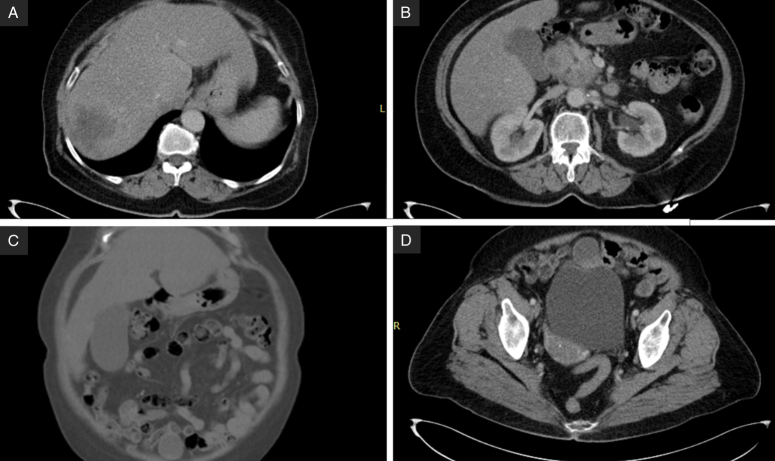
Computed Tomography scan, (A) Axial section: The seventh hepatic segment mass appearing hypodense, (B) Axial section: 53×77 mm mass in the hepatic hilum and infiltrating the head of the pancreas and duodenum, (C) coronal section: shows the hepatic helium mass, (D) Axial section: shows a hypodense nodule on the anterior wall of the bladder.
